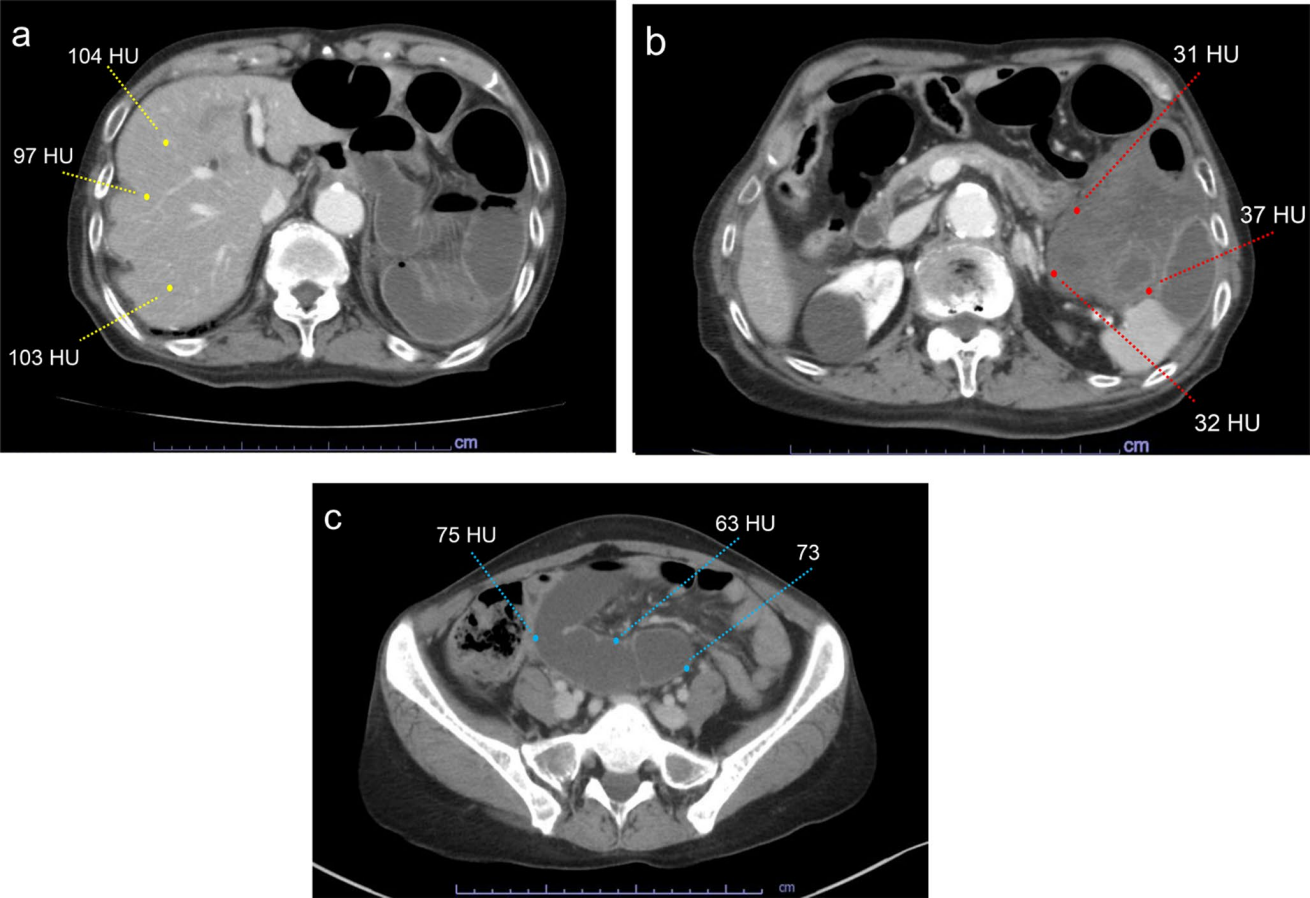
Evaluation of SBO using the CT ratio in the equilibrium phase of the CT images. a: An example of randomly selecting three small points of the liver parenchyma. b: An example of randomly selecting three small points of the least enhanced intestinal wall. The mean of the CT values was 33.3. This was an example of the CT attenuation ratio below 0.4. c: An example of randomly selecting three small points of the least enhanced intestinal wall. The mean of the CT values was 70.3. This was an example of the CT attenuation ratio above 0.4
